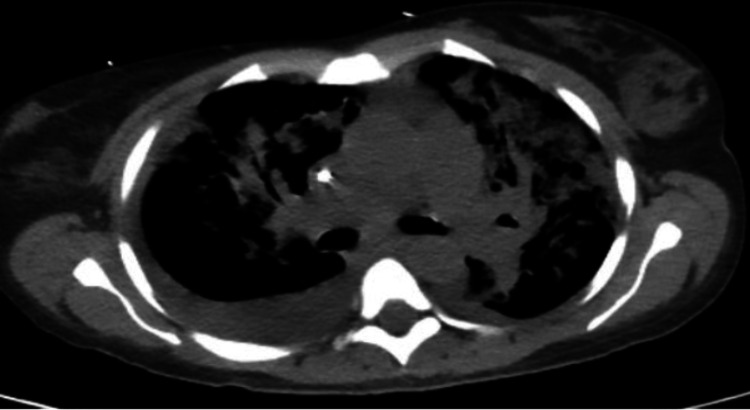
Chest CT demonstrating extensive bilateral airspace disease and pleural effusions
